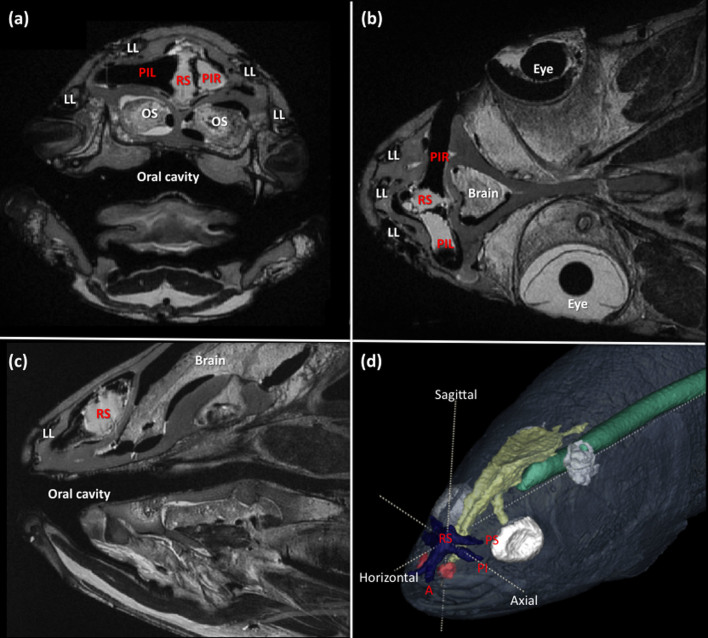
Morphology of the rostral organ electric sense in the extant coelacanth, *L. chalumnae*. MRI grey-scale data showing (a) axial, (b) horizontal, and (c) sagittal plane cross-sectional slices through the head at the location of the rostral organ. (d) 3D reconstruction from image segmentation of MRI grey-scale data showing in situ rostral organ morphology (depicted in blue). A selection of rostral organ structures have been annotated following the terminologies of Bemis & Hetherington^14^ with abbreviations provided below. The rostral sac (RS) resides within the median rostral cavity in the ethmoid portion of the chondrocranium. It contains all of the electrosensory epithelia and comprises a system of crypts that are invaginated into the rostral sac tissues. Three pairs of tubules radiate out from the rostral sac to pores opening on the surface of the snout. These bilaterally paired tubules comprise the anterior (A), posterior inferior (PI), and the posterior superior (PS) tubules. Note that in life, the spaces within the rostral sac and tubule systems are filled with a gelatinous substance that was stripped away as a result of tissue fixation and preservation. The thin layer of fatty tissue that surrounds the tubules and rostral sac in life has similarly been lost. Abbreviations: A, anterior tubule; LL, lateral line canal; OS, olfactory structures; PI, posterior inferior tubule; PIL, left posterior inferior tubule; PIR, right posterior inferior tubule; PS, posterior superior tubule; RS, rostral sac.
